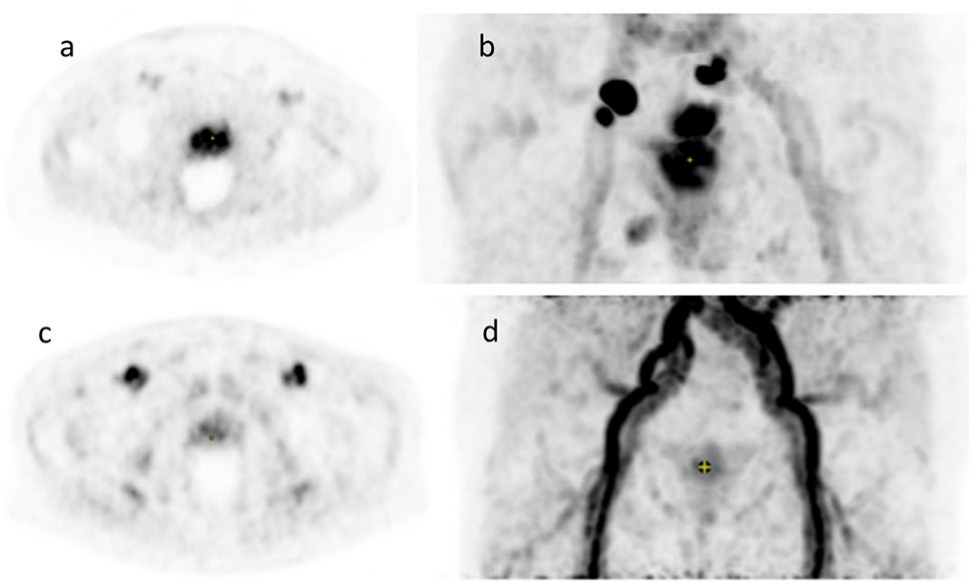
Visual comparison of two patients shows association between SUVmax levels and tumor stage. In the upper row (a, b) SUVmax was 27.2 and MTV 38.1 while in the lower row (c, d) SUVmax was only 9.6 and MTV 26.0. Patient on the upper row also presented with several pelvic lymph node metastasis, visible in MIP mode (b).
